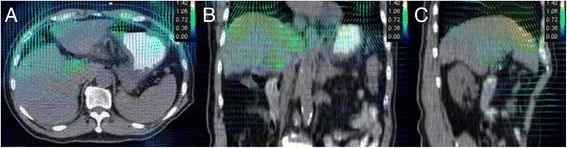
Deformation fields for tumor and liver from contrast-enhanced 3D CT images to different respiratory phases of 4D CT images. A. axial view. B. coronal view. C. sagittal view in CT_00_ respiratory phase. The variation of arrows and color show the vector fields on the axial, coronal and sagittal slices.
